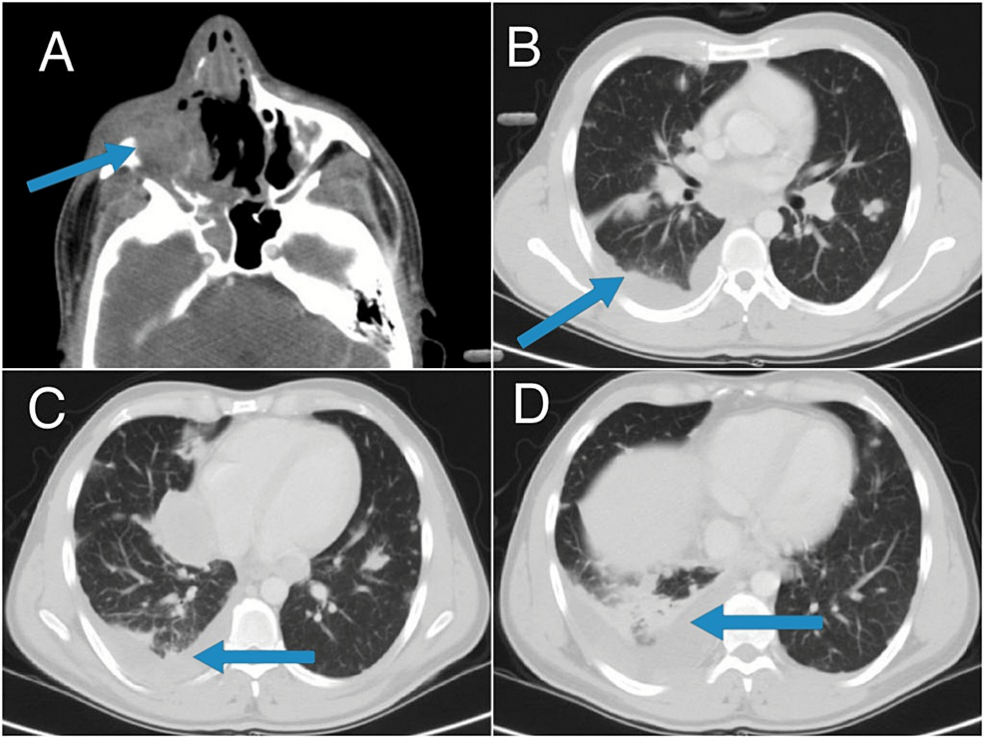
Restaging CT during radiotherapy showing via the arrow in (A) significant interval decrease in the bulky lobulated soft tissue mass with its epicenter in right maxillary sinus. The arrows in (B), (C) and (D) show progressive disease by virtue of interval development of pulmonary, pleural, and hepatic metastasis along with metastatic mediastinal lymphadenopathy
